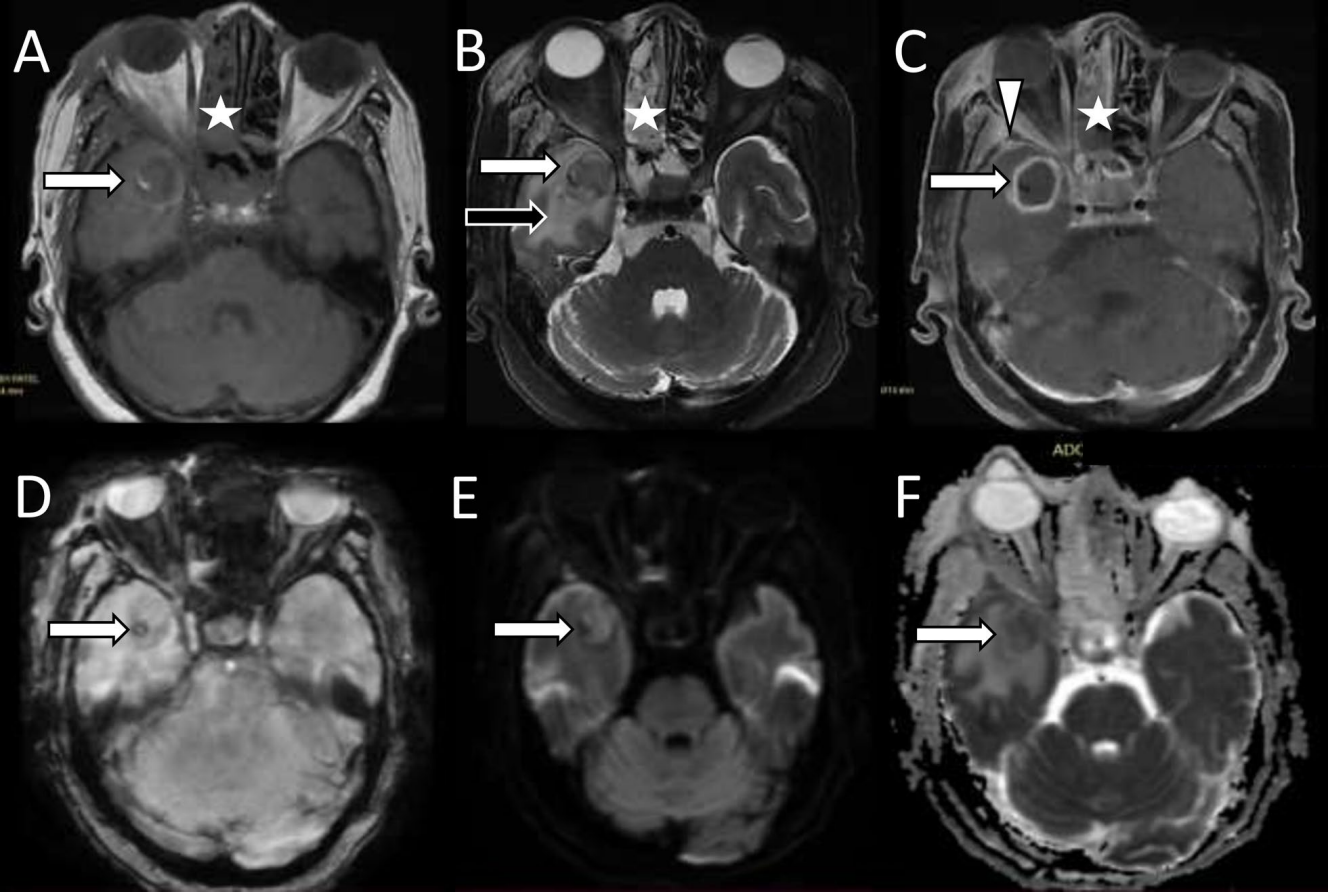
Fungal cerebral abscess. Axial T1-weighted image (A) shows a well-defined isointense lesion in the right temporal lobe (white arrow). Axial T2-weighted image (B) shows low signal (white arrow) with surrounding edema (black arrow). Contrast-enhanced axial T1-weighted fat-saturated image (C) shows ring enhancement of the lesion (white arrow) with associated meningeal enhancement (arrowhead). Susceptibility-weighted image (D) shows foci of blooming (white arrow) inside the lesion (likely fungal elements). Diffusion-weighted and apparent diffusion coefficient (ADC) images (E, F) show diffusion restriction with low ADC signal (white arrows). Note the soft tissue in right ethmoid sinuses suggesting their involvement (white stars in images A–C)
